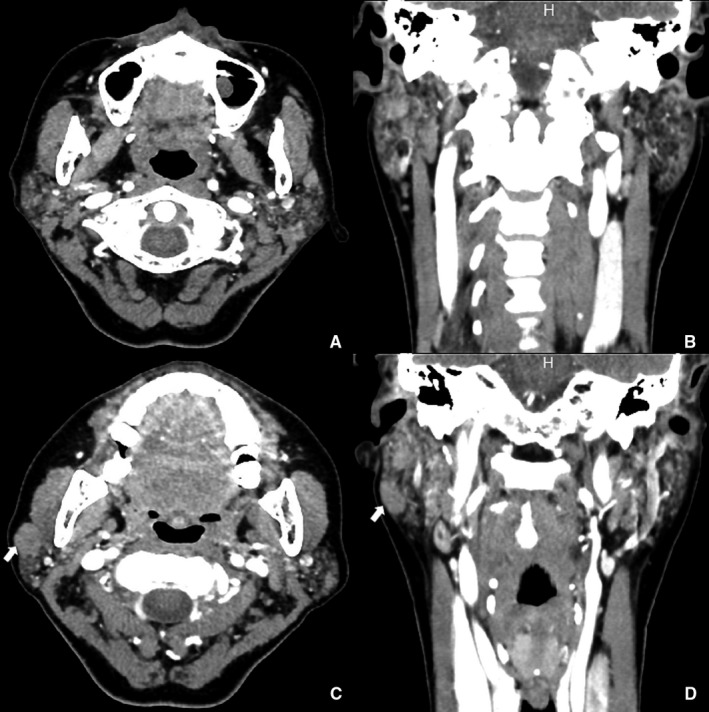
Enhanced computed tomography suggested MALT lymphoma in the right parotid gland (57‐year‐old female). Bilateral parotid glands were uneven with spotted high‐density focus, considered as a lymphoepithelial lesion or SS (A, B). Multiple solitary masses were present; the larger one (1.7 × 1.2 cm) was on the right (C, D)
